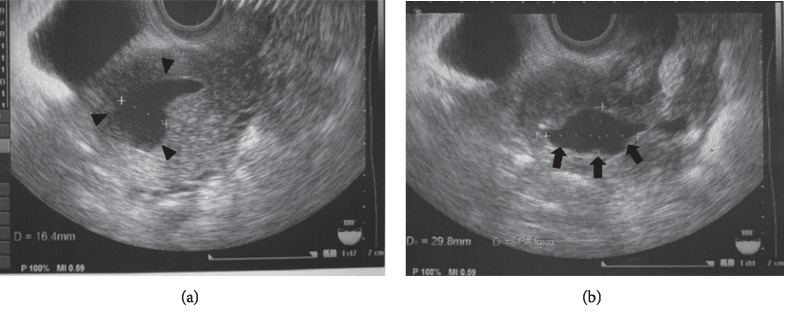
Transvaginal ultrasound on postoperative day 62 showing dilated uterine cavity ((a), arrowheads). The cystic echo observed on day 34 still exists but decreased in size ((b), arrows, 29.8 mm × 15.1 mm).
